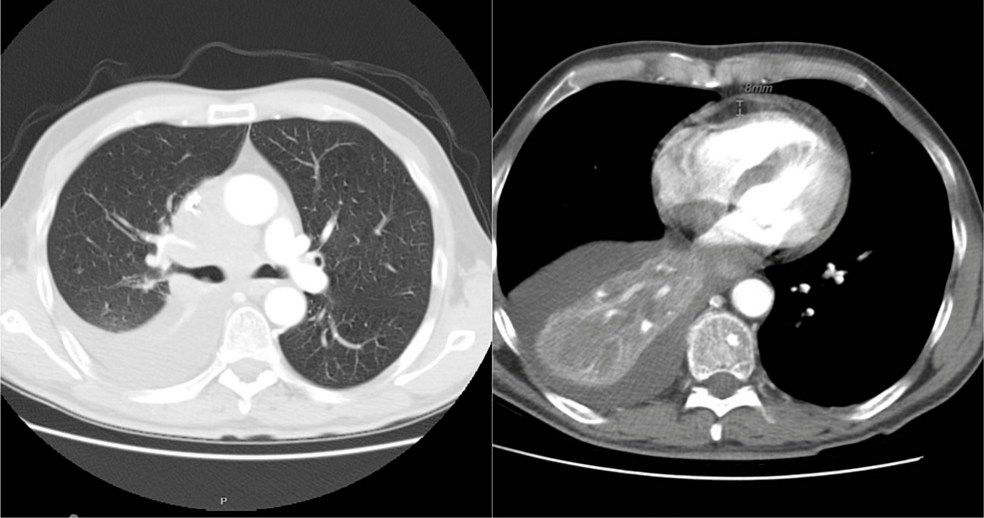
A CT of the chest showed pleural effusion (A) and PE on the right side, as well as a right infra-hilar mass, mediastinal adenopathy, and hilar adenopathy (B)
